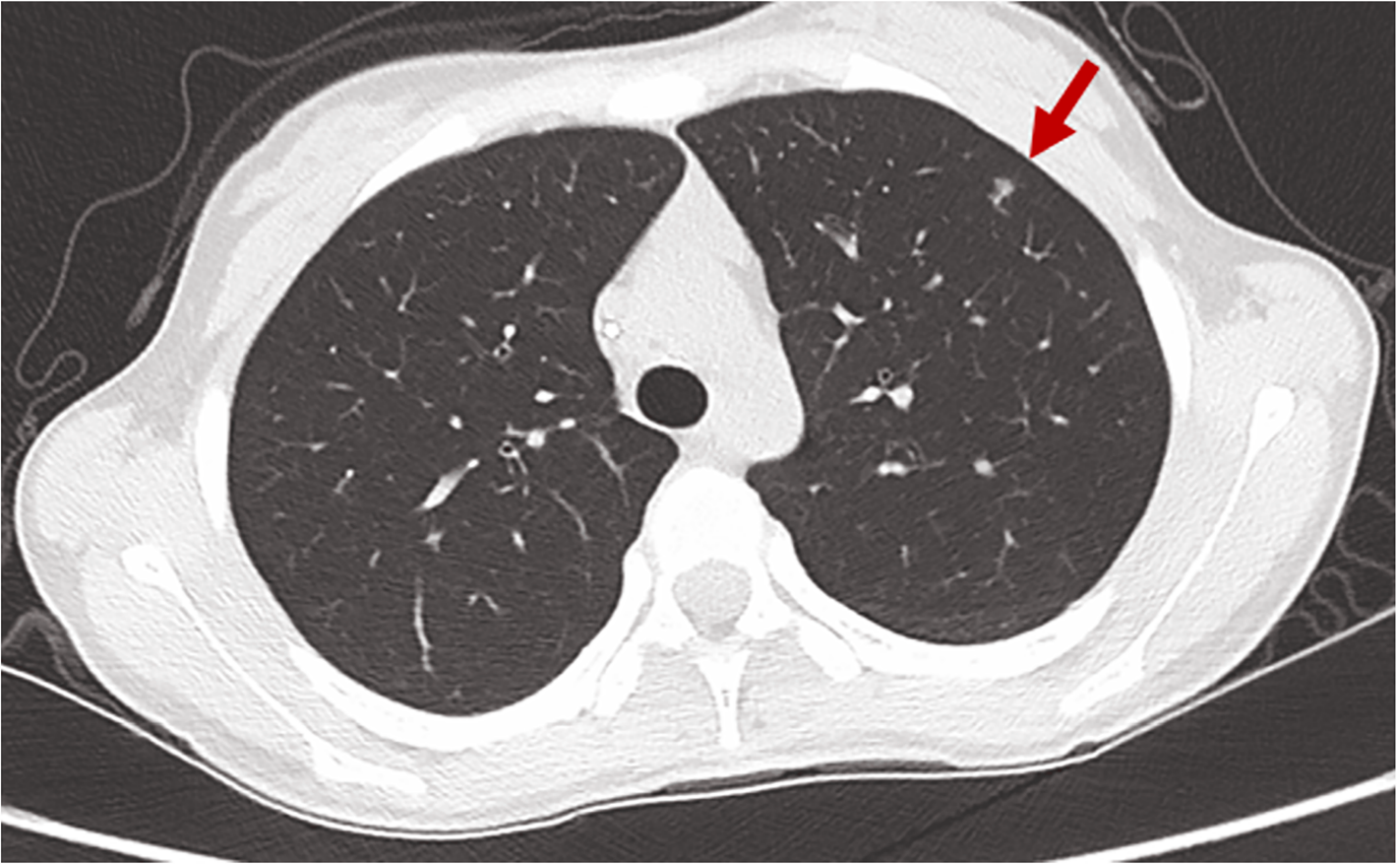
A missing nodule shown on chest CT (red arrow) before metastasectomy. (It was not resected later. CT = computed tomography)
